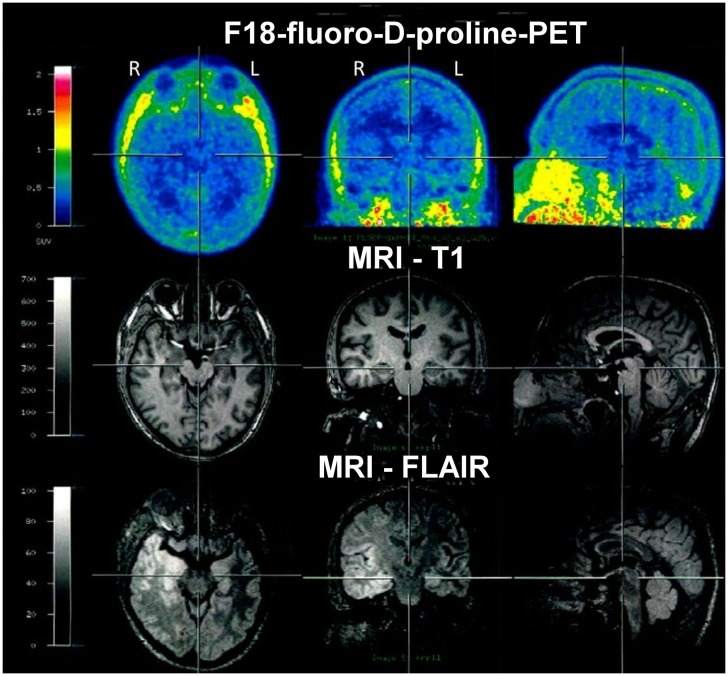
F18-fluoro-d-proline-positron emission tomography (PET) in March 2012 showed no abnormal tracer accumulation. Structural MRI again showed bitemporal hyperintensities, however, at that time clearly more marked within the right hemisphere.
